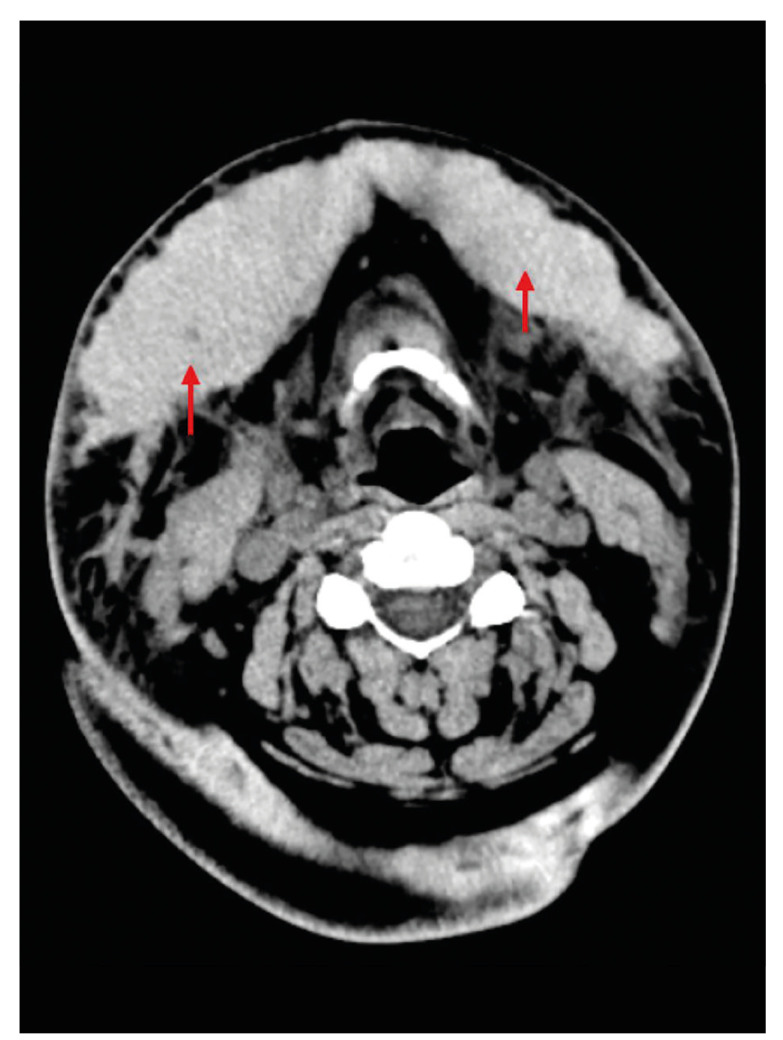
Computed Tomography (CT) showing large subcutaneous hematoma.
